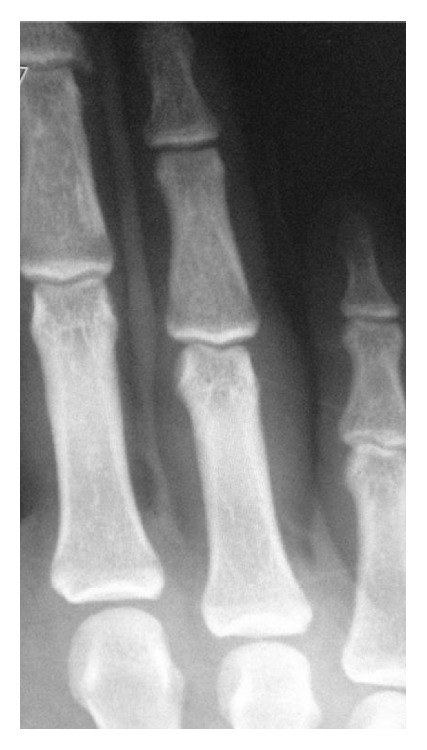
At the end of 6 months, X-ray showing joint congruity.
